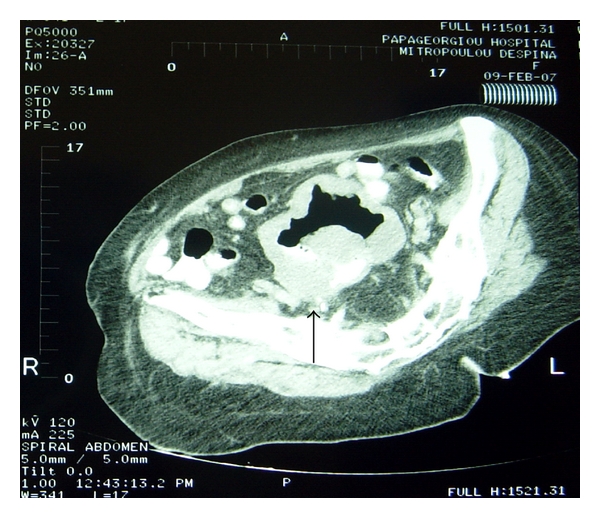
Preoperative CT examination of the patient.
